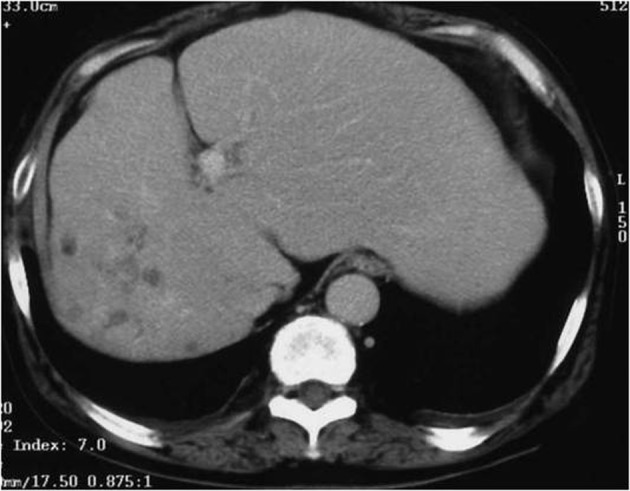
Computed tomography with contrast medium (portal phase). The presence of abscess was suspected at the right lobe of the liver.
